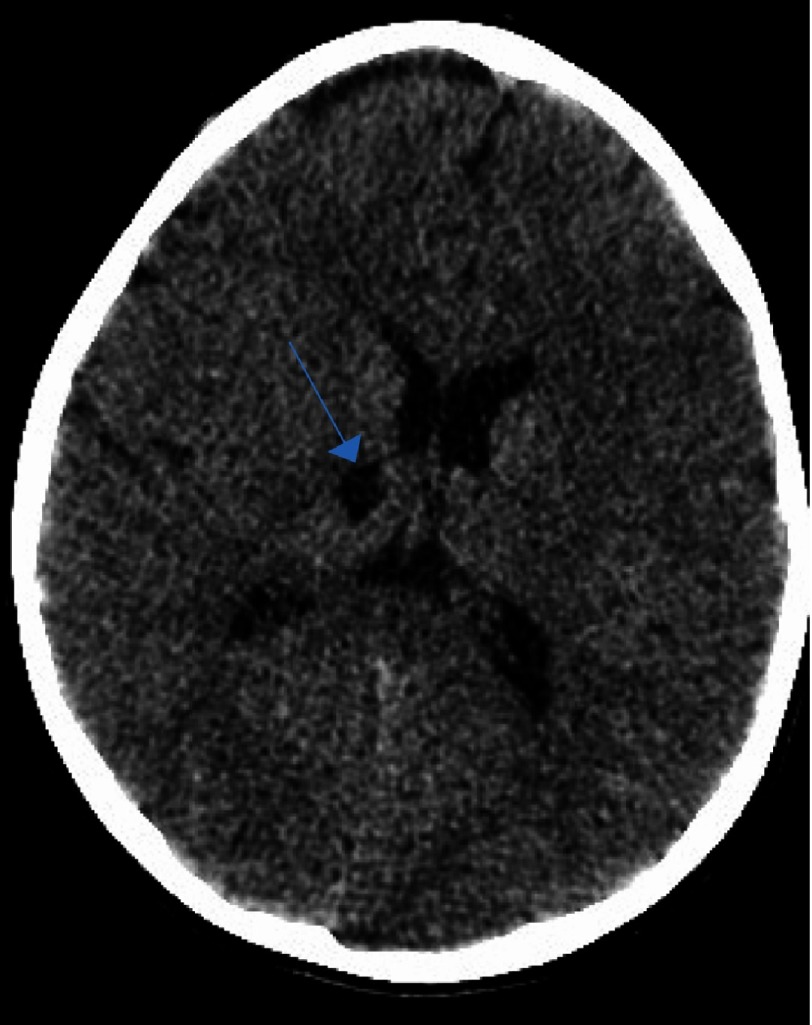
CT scan after the first ischemic insult. Note the hypointensity signal area at the limit between the genu and the posterior limp of the internal capsule of the right basal ganglia (arrow).
